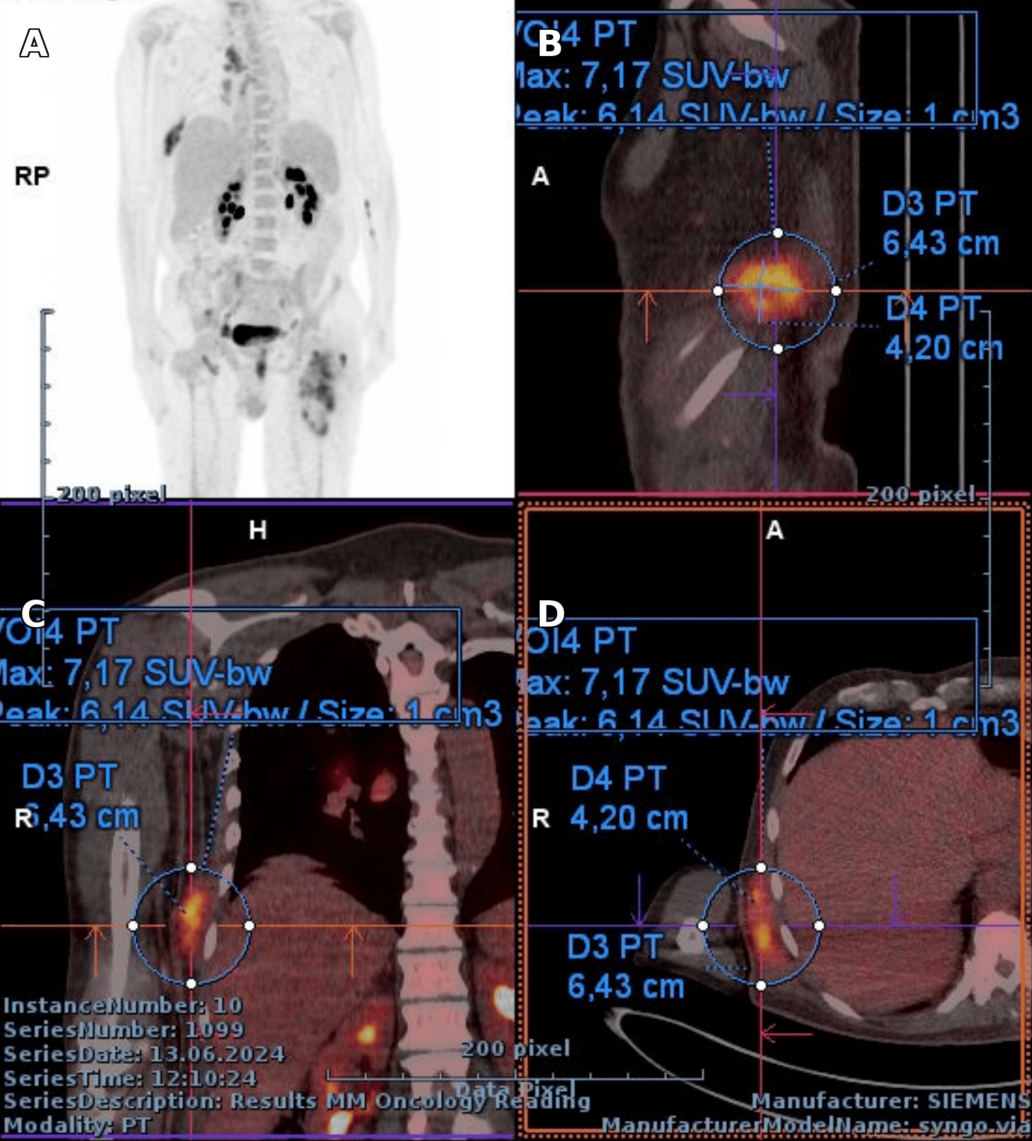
PET/CT of the thorax: (A) MIP showing thoracic hypermetabolic foci; (B) Sag slice with lesion 6.43 cm (D3 PT); (C) Cor slice with SUVmax 7.17 (VOI4 PT); (D) Ax slice confirming lesions 6.43 cm (D3 PT) and 4.20 cm (D4 PT)
